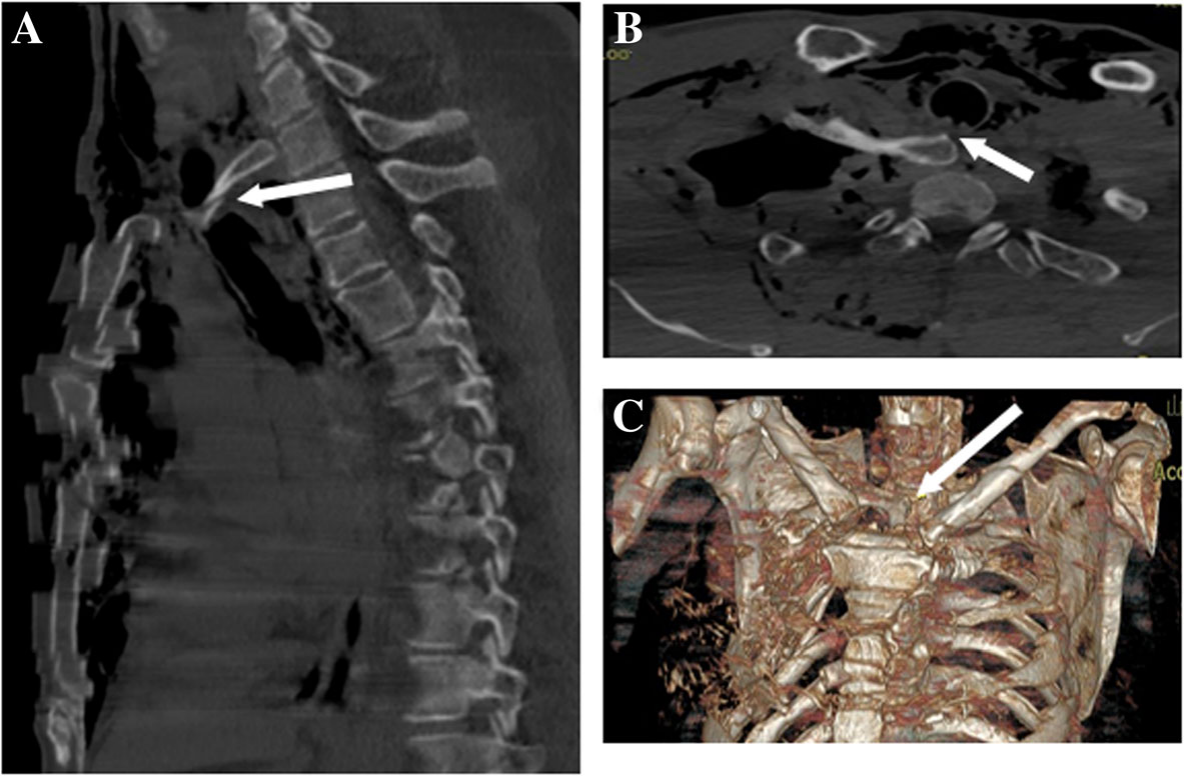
CT scans of multi-slice technique showing the tracheal laceration secondary to the dislocation of right first rib. a Sagittal CT image of the chest. b Axial CT image of the chest. (C) Volume rendering of thorax
